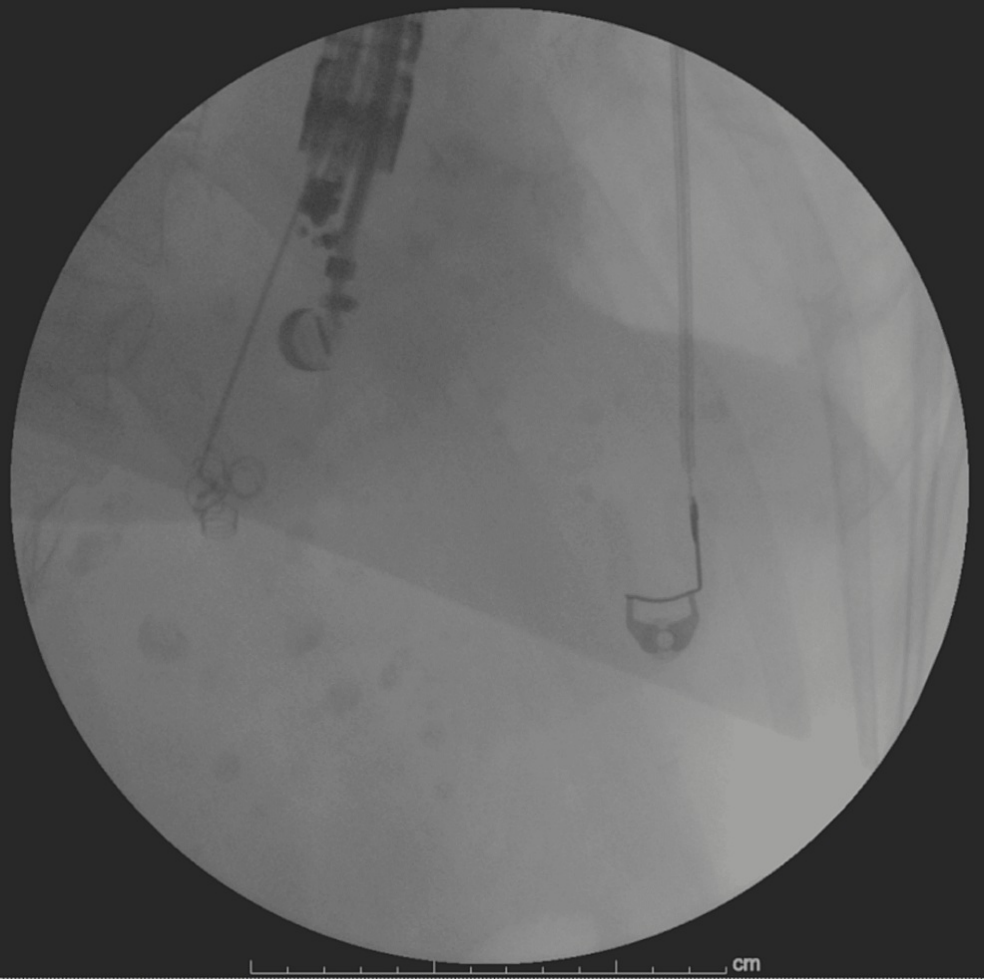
Fluoroscopic image of EUS-guided coil placement EUS: endoscopic ultrasound
